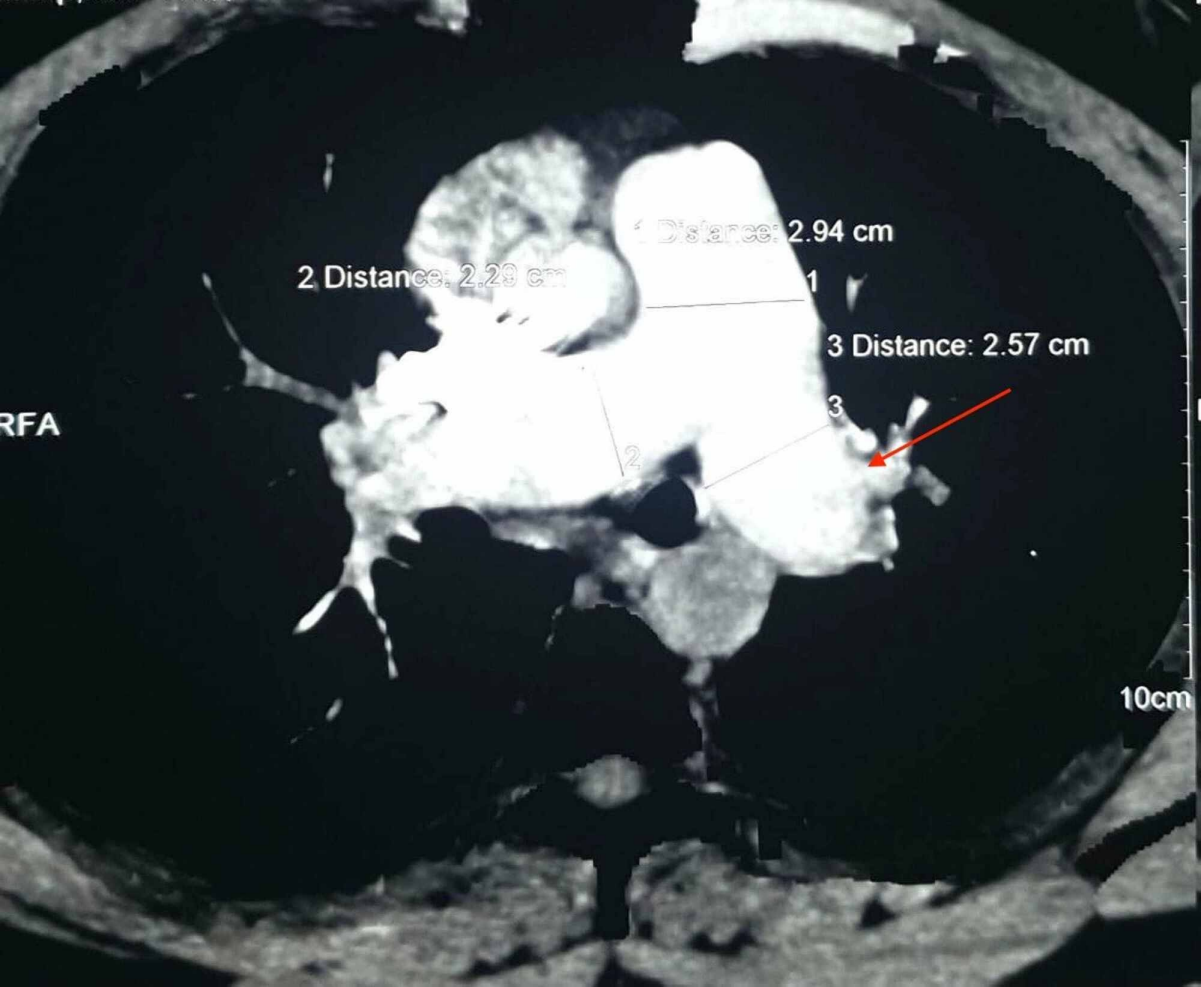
Axial section of CTPA showing minimal reduction in size and extent of filling defect in the left main pulmonary artery compared to previous CTPA scan. CTPA - Computed tomography pulmonary angiogram
